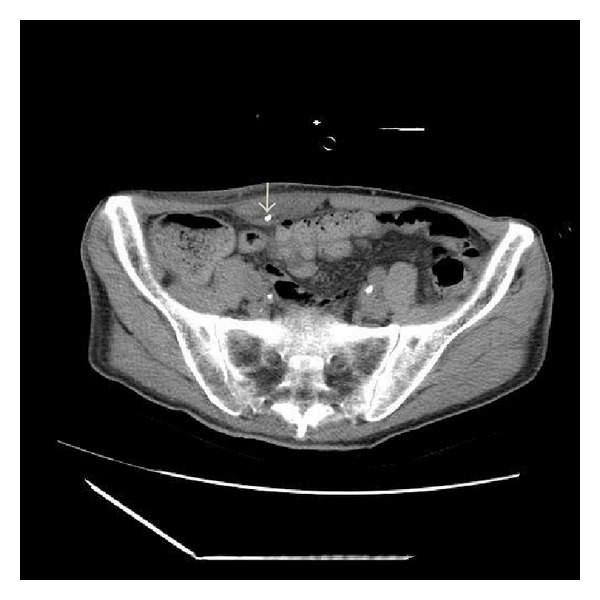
Axial computed tomography reveals the catheter in the right inferior epigastric vein as well as swelling of the right lower rectus abdominis muscle and adjacent subcutaneous tissue.
